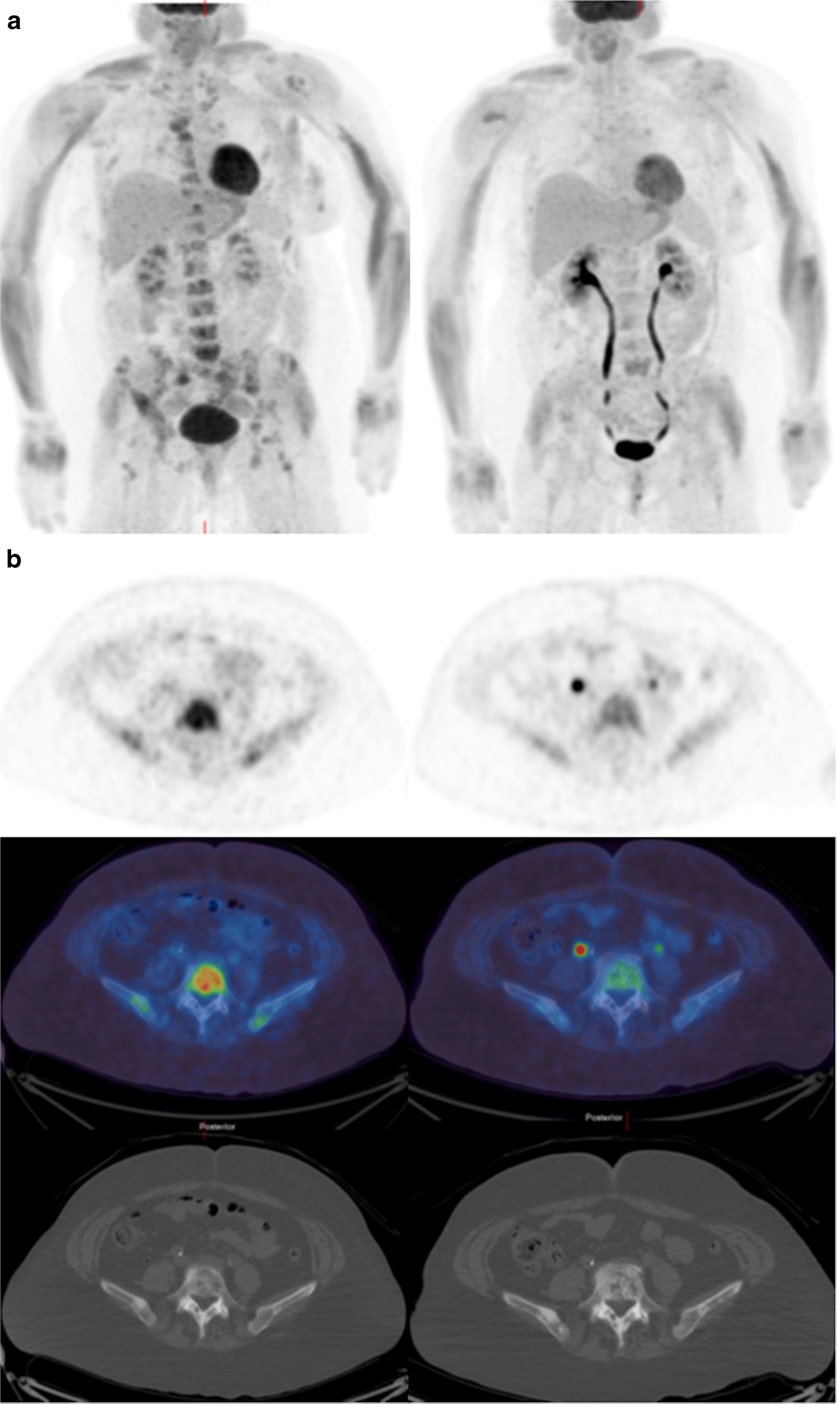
a FDG PET MIP and b corresponding transaxial images [PET (*top*), fused PET/CT (*middle*), and CT (*bottom*)] of a lesion in L5 at baseline (*left*) and 8 weeks (*right*) in the same patient as Figs. 2 and 4. SUV_max_ of the L5 vertebral lesion decreased from 9.9 to 4.9
